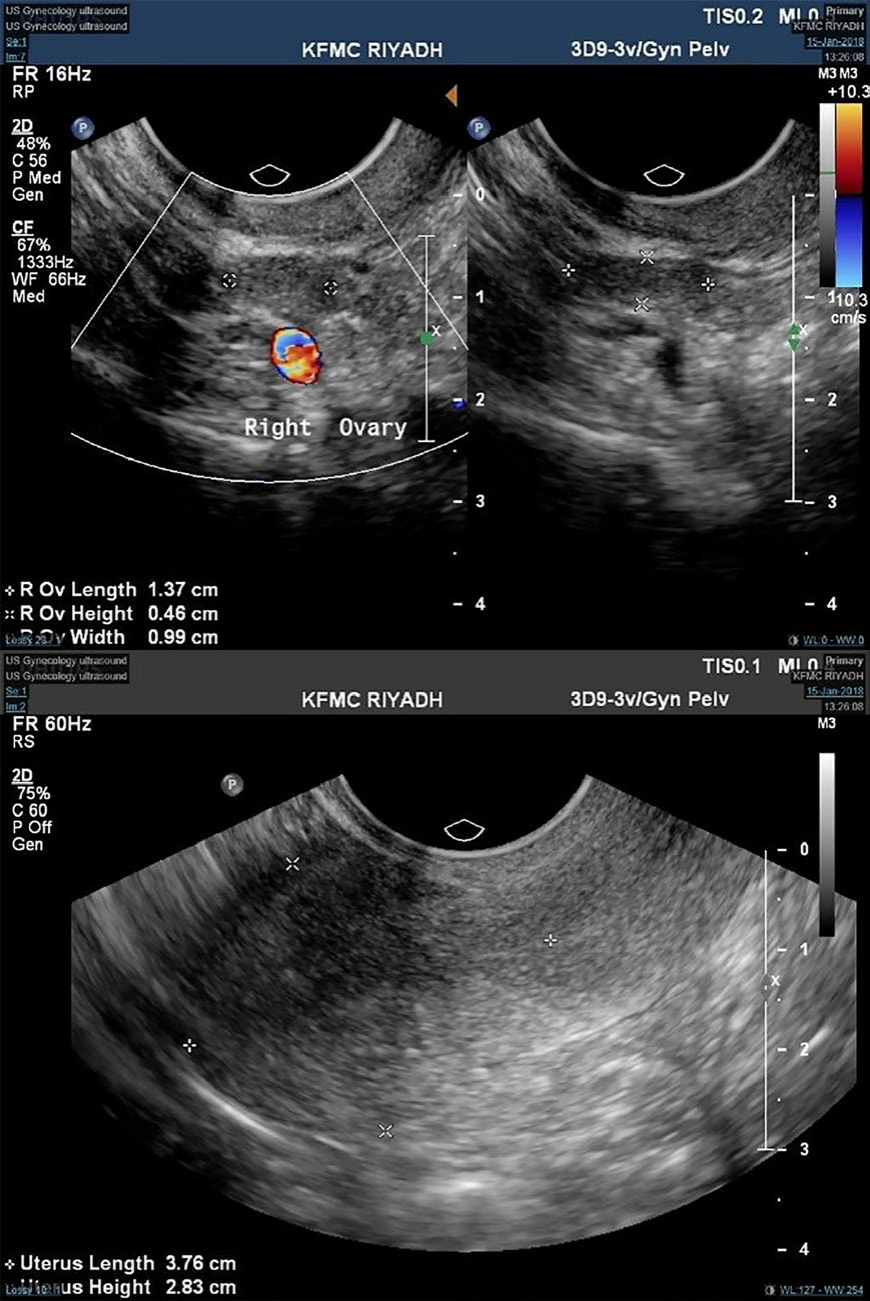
Ultrasound showing streak ovaries with no follicles and a normal uterus
